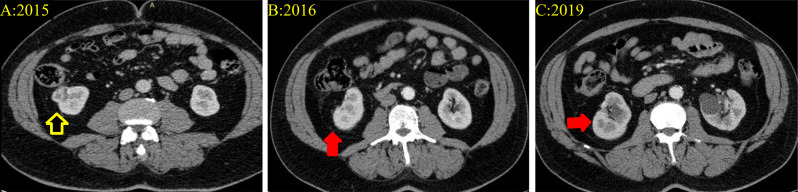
Selected CT scans at different times in which (A) is showing an exophytic rounded hyperenhancing cortical lesion in the lower pole of the right kidney measuring about 2.7 × 2.2 × 2.1 cm in in AP x CC x TS diameters respectively (black arrow with yellow highlight) consistent with the partially resected renal cell carcinoma of clear cell subtype. (B & C) demonstrating the site of Partial nephrectomy with clear surgical site (Red arrows)
